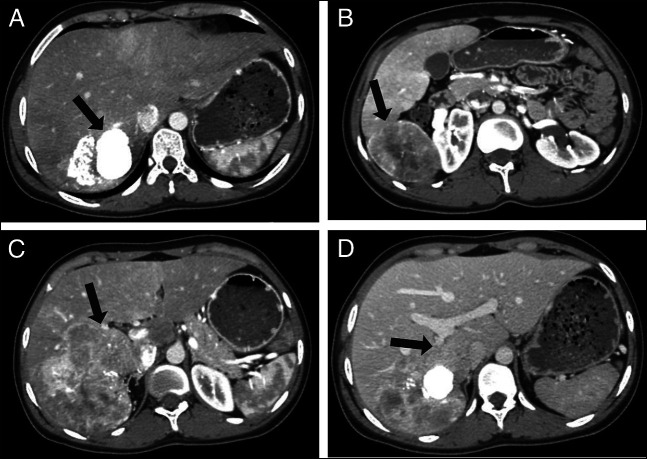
Abdominal MRI with contrast. (A, B) T1-weighted axial sections showing a large CNSET (white arrow) involving the right lobe of the liver. (C) T2-weighted axial section showing the tumor with a central scar. (D) T2-weighted image showing an exophytic component of the tumor on the coronal section. CNSET, calcifying nested stromal-epithelial tumor; MRI, magnetic resonance imaging.
